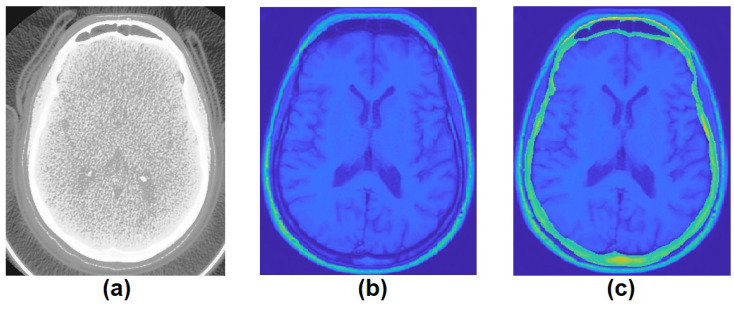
(a) CT scan, (b) MRI, and (c) STL model extracted from CT scan overlapped in MRI.
